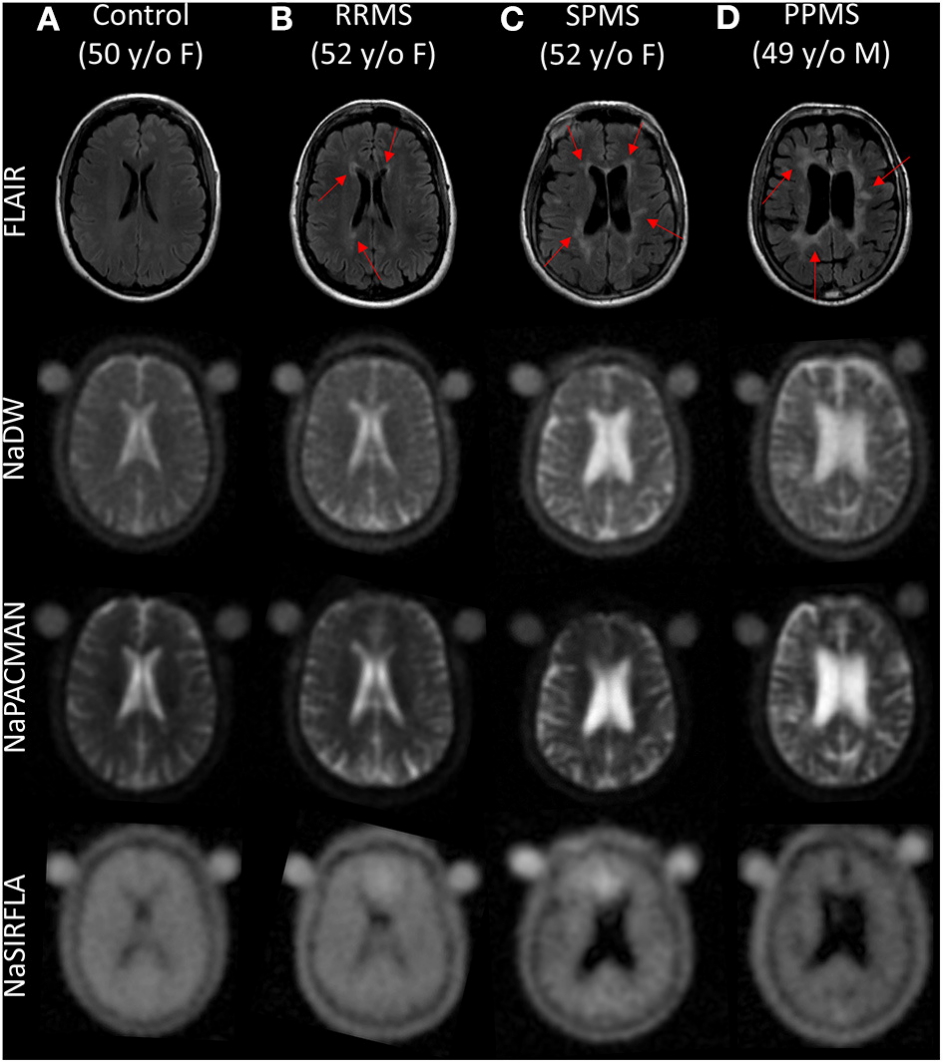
Qualitative axial image comparison of (A) a healthy control and (B–D) three MS subtypes for FLAIR, NaDW, NaPACMAN, and NaSIRFLA. Regions of hyperintensity on FLAIR (red arrows) have visibly greater sodium signal compared to NAWM on NaDW and NaPACMAN (hyperintense), where NaPACMAN shows the greatest lesion contrast. Lesion sodium intensity on NaSIRFLA is difficult to visually distinguish from NAWM, but is slightly hypointense. Note that NaSIRFLA images display an artifact of hyperintensity in the frontal sinus cavity region due to off-resonance effects.
